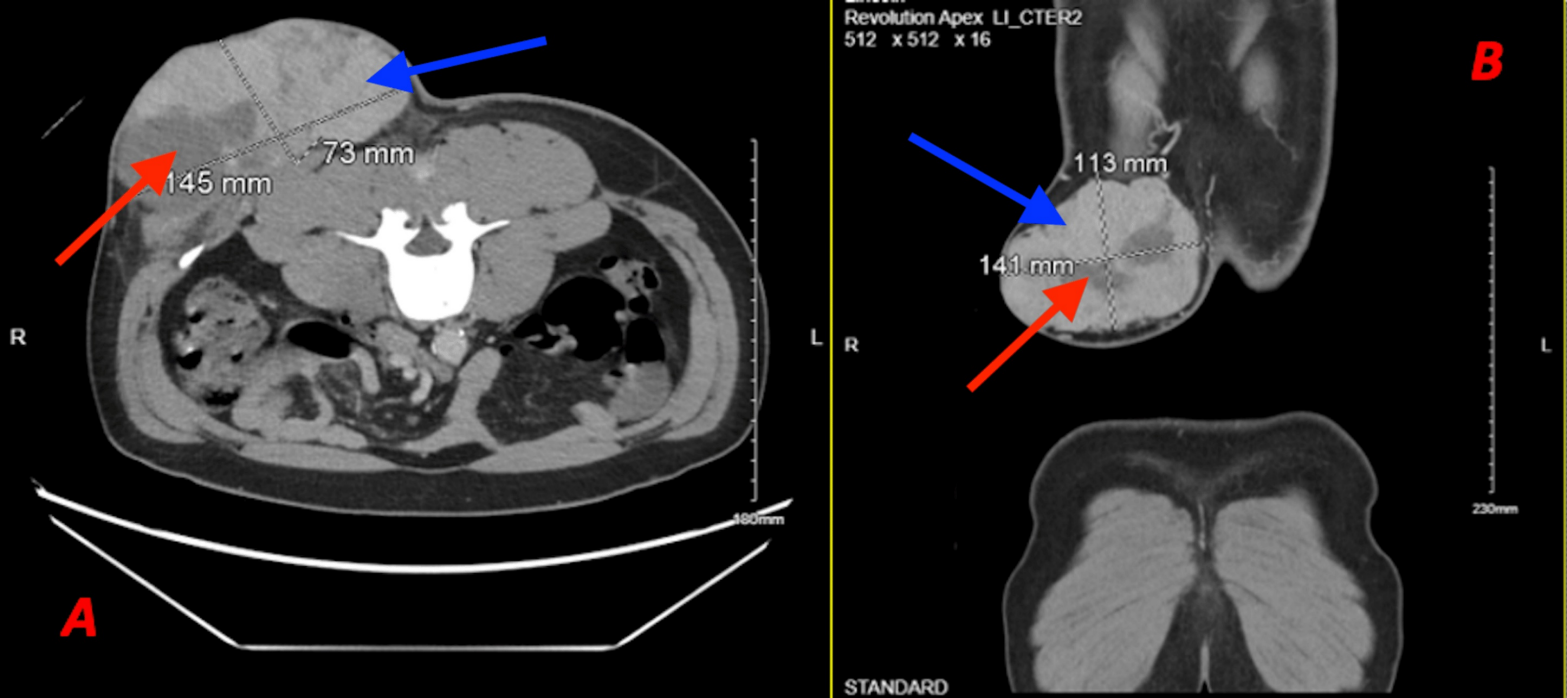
CT Scan Taken on the Day of Presentation Axial (A) and coronal (B) CT scan sections, which showed the size of the mass on the patient's back on the day of his presentation to the emergency department. Two distinct densities are appreciable within the tumor, denoted by blue (less dense) and red (more dense) arrows. CT: computed tomography
